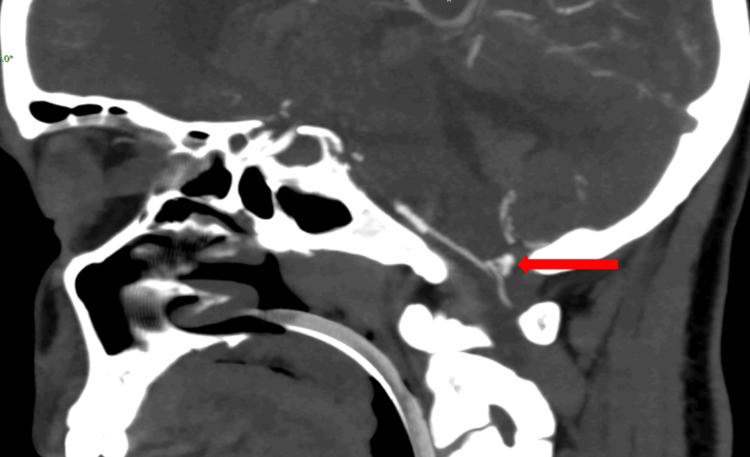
Computed tomography angiography brain showing left posterior inferior cerebellar artery aneurysm.
